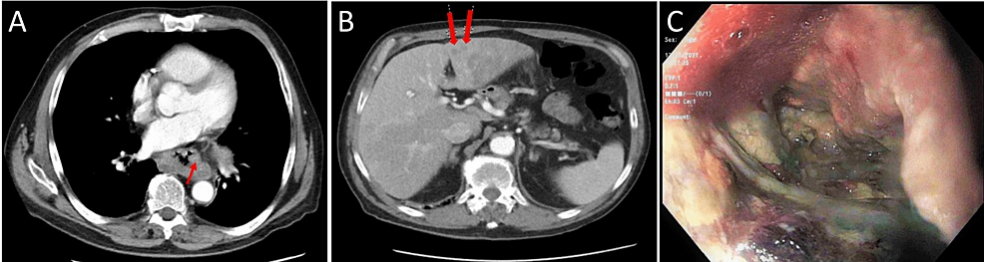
Case 2 images A: CT abdomen showing irregular thickening of the distal esophagus and neoplastic infiltration and necrosis along the lateral walls of the esophagus (red arrow). B: CT showing multiple hepatic metastases (red arrows); the largest was 18.4 mm (not shown). C: EGD showing a fungating circumferential, necrotic, distal esophageal ulcer with stigmata of recent bleeding. EGD: esophagogastroduodenoscopy
